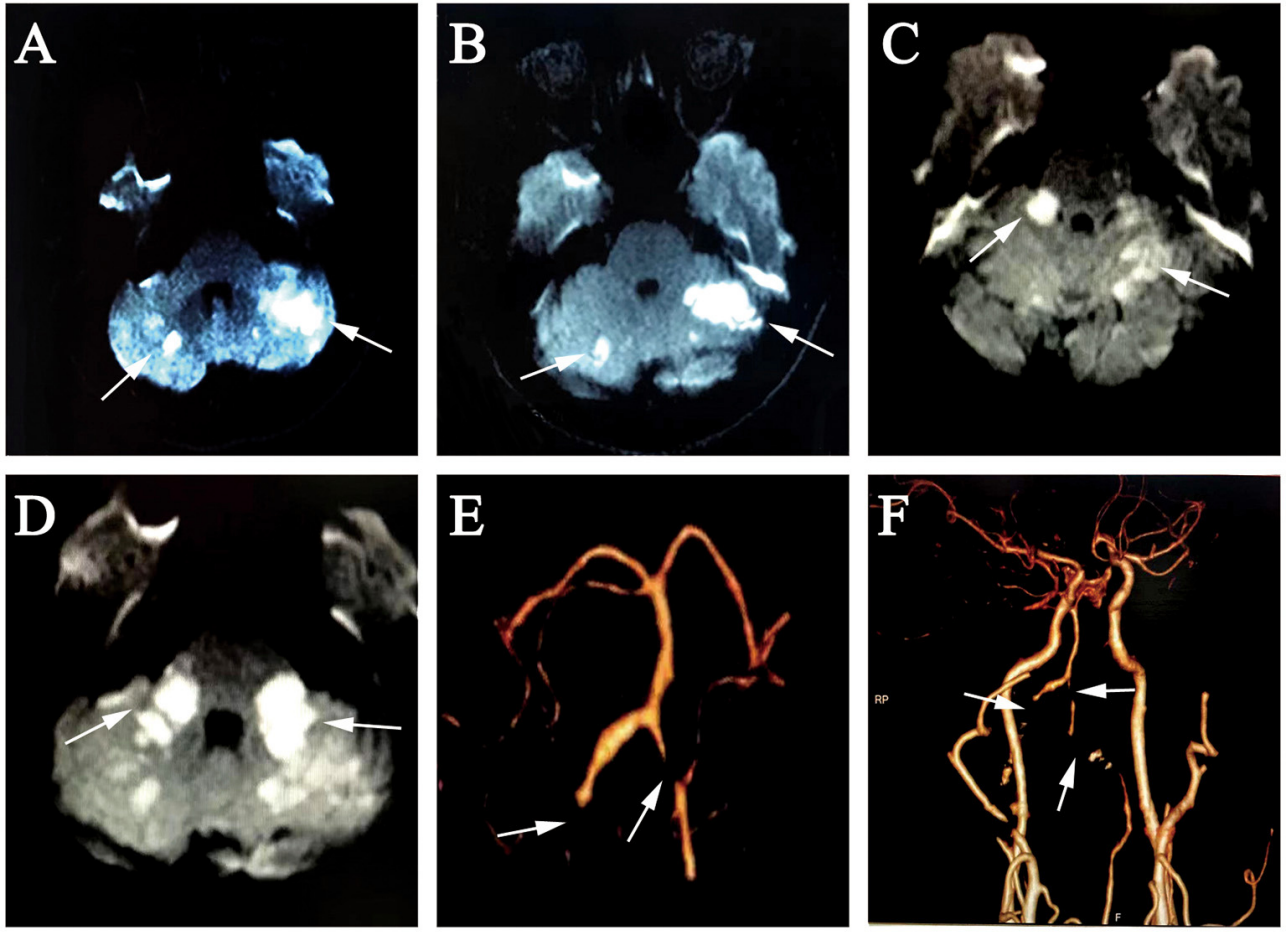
(A, B) 3rd day, diffusion-weighted MRI showed hyperintense foci at the bilateral cerebellum; (C, D) 10 days later, diffusion-weighted MRI showed new hyperintense foci at the bilateral MCP; (E, F) brain and neck CTA revealed occlusion of the bilateral VA V3 segment, proximal V4 segment and bilateral AICA, severe stenosis of the distal left VA V4 segment. MRI, magnetic resonance imaging; MCP, middle cerebellar peduncle; CTA, computed tomography angiography; VA, vertebral artery; AICA, anterior inferior cerebellar artery.
